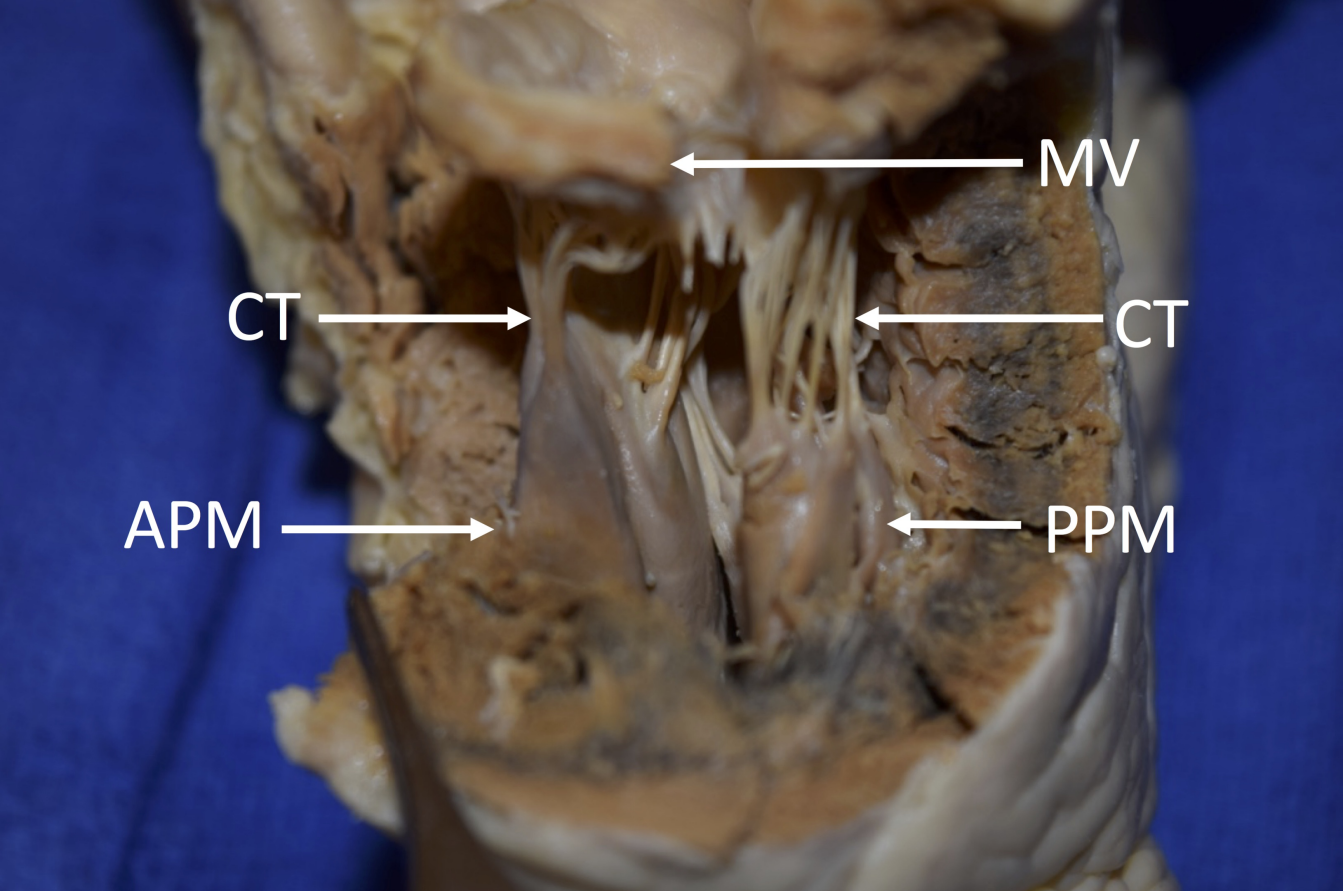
Lateral view of the left ventricle. APM; anterior papillary muscle, CT; chordae tendinae, MV; mitral valve, PPM; posterior papillary muscle
